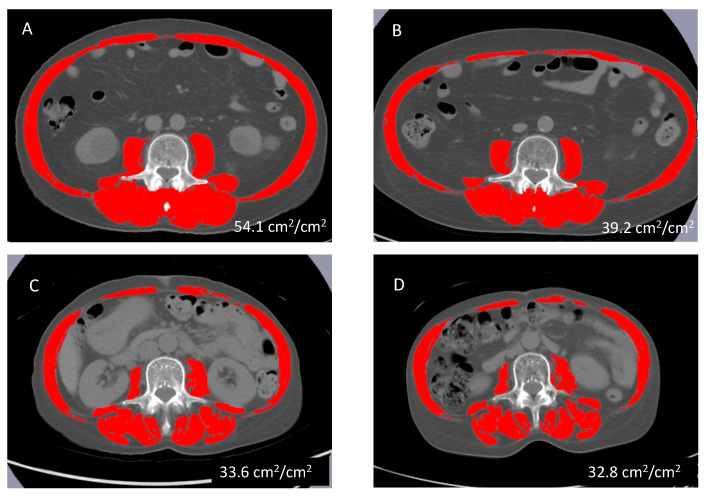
Axial CT image of the third lumbar spine region with skeletal muscles highlighted in red. CT images of a 70-year-old man with non-SMD before (A) and after 25 cycles of AZA (B); CT images of a 68-year-old woman with SMD before (C) and after 11 cycles of AZA (D). Abbreviations: CT, computed tomography; SMD, skeletal muscle depletion; AZA, azacitidine.
